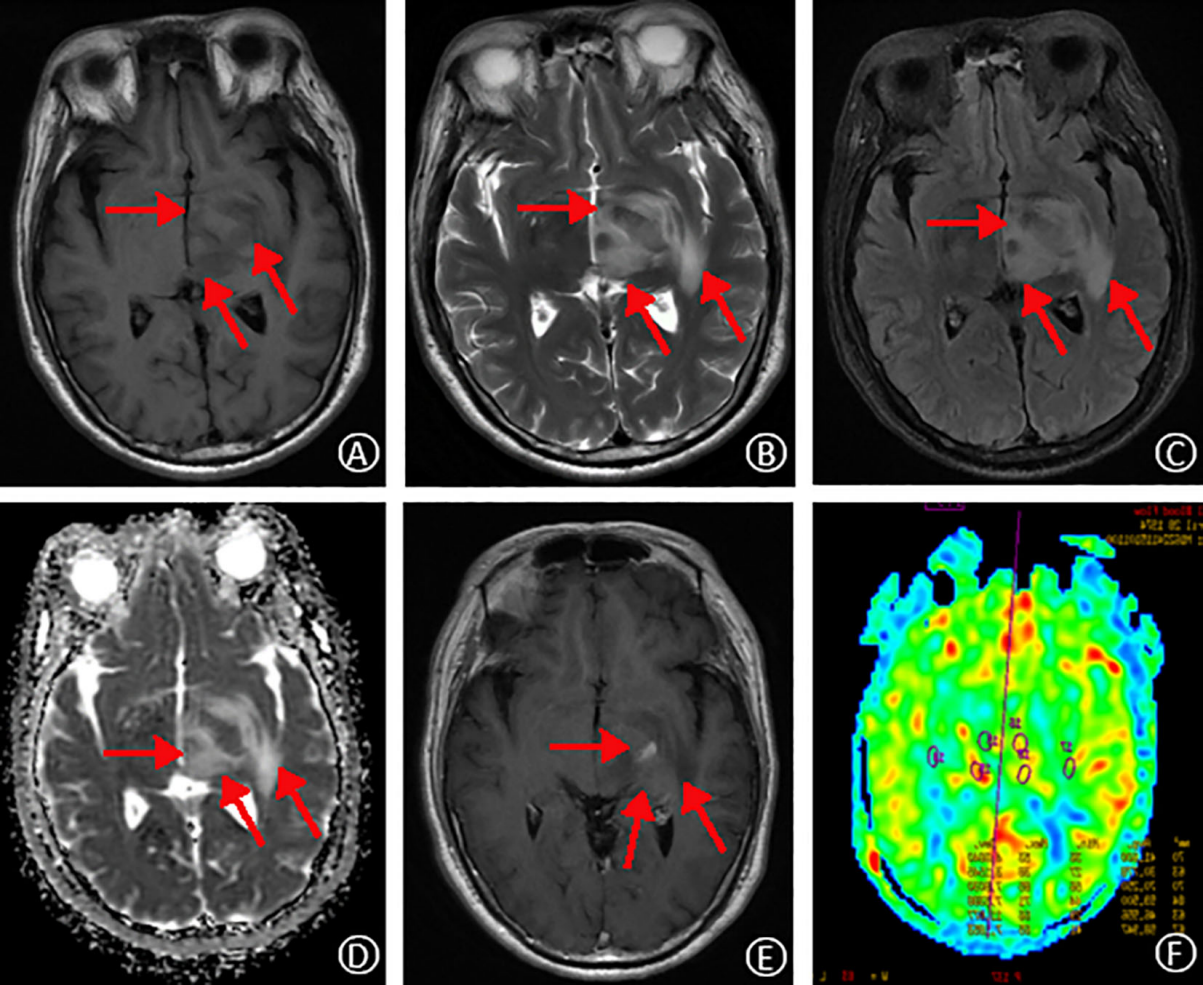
MRI findings on initial admission. T_1_-weighted and T_2_-weighted images, respectively, show slightly prolonged signal intensity in the left temporal lobe, thalamus, and basal ganglia regions (A, B). Fluid-attenuated inversion recovery (FLAIR) sequence reveals high signal intensity in the same regions (C). Apparent diffusion coefficient (ADC) map demonstrates mildly elevated signal intensity (D). Contrast-enhanced T_1_-weighted image shows focal areas of speckled and striated enhancement within the left temporal lobe, thalamus, and basal ganglia (E). Arterial spin labeling (ASL) perfusion map reveals increased perfusion in the corresponding areas of abnormal signal intensity (F).
